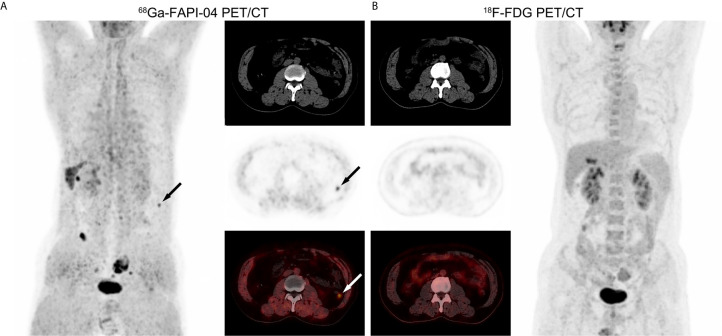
PET/CT images in a 51-year-old male patient with poorly-differentiated HCC and regional lymph node metastasis. (A)
